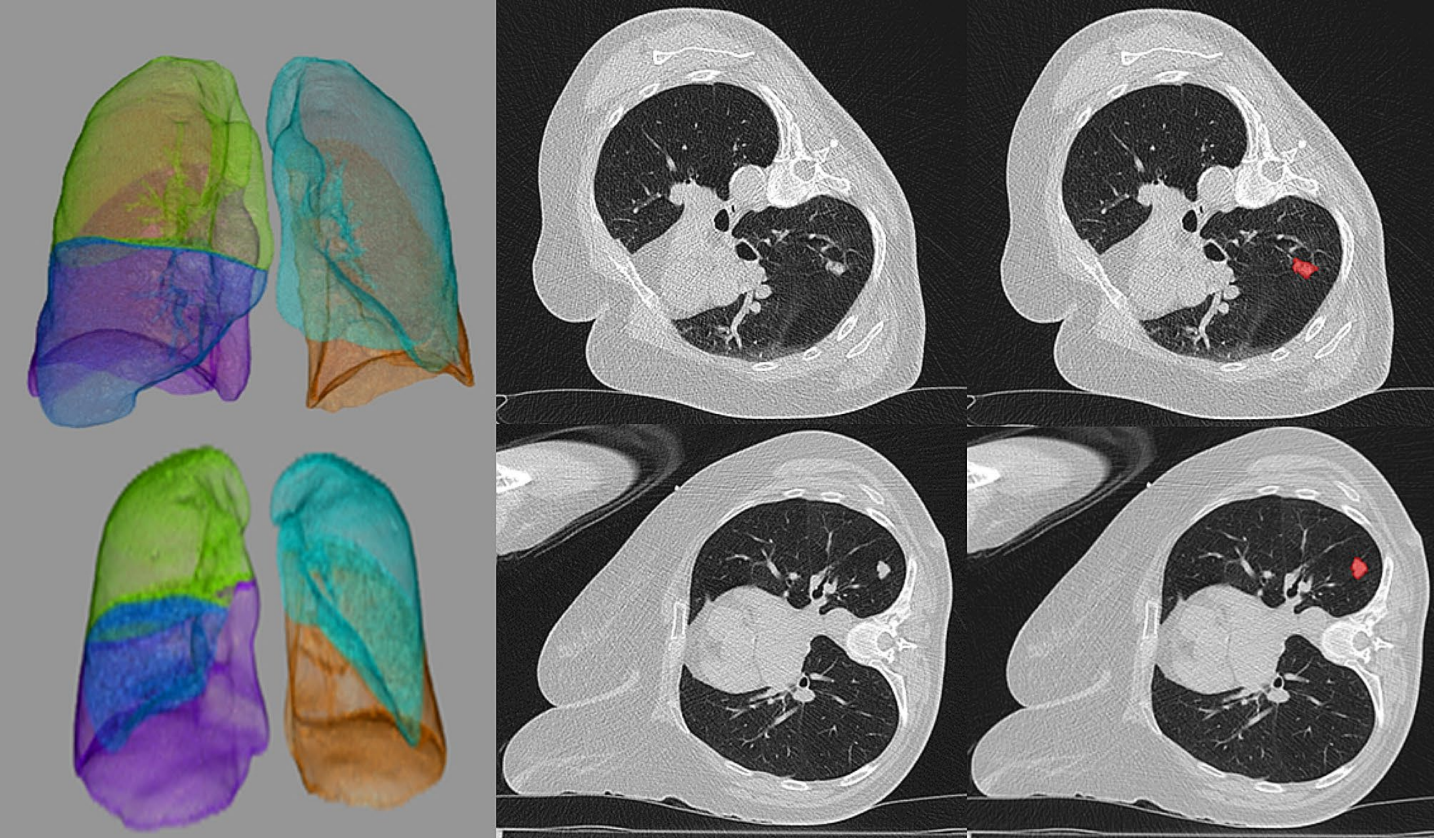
Representative images of the patient sample. First row: segmentation of the lung in order to achieve automated emphysema scoring. Second row: representative slice of the CT Thorax planning scan demonstrating the biopsied lung lesion. Third row: drawn region of the interest (in red) of the biopsied lung lesion. First line: 71-year-old male patient, right-elevated position, no pneumothorax after biopsy, biopsy successful (non-malignant lesion). Second line: 67-year-old female patient, right-lateral position, pneumothorax after biopsy, biopsy successful (metastatic lesion)
